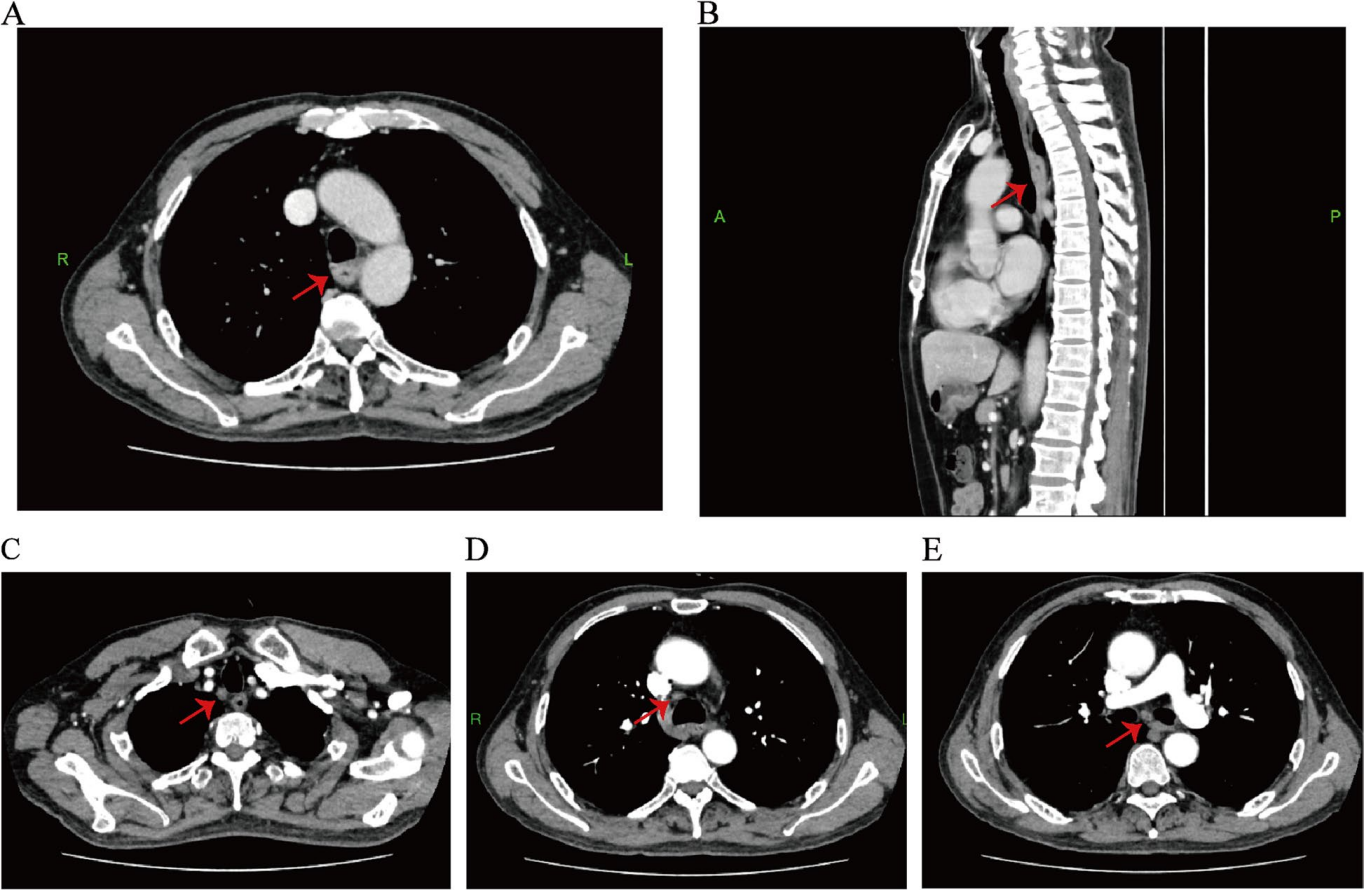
Pre-treatment examination: computed tomography. A, B The red arrows indicate the esophageal cancer lesions. C–E The red arrows indicate the swollen lymph nodes
